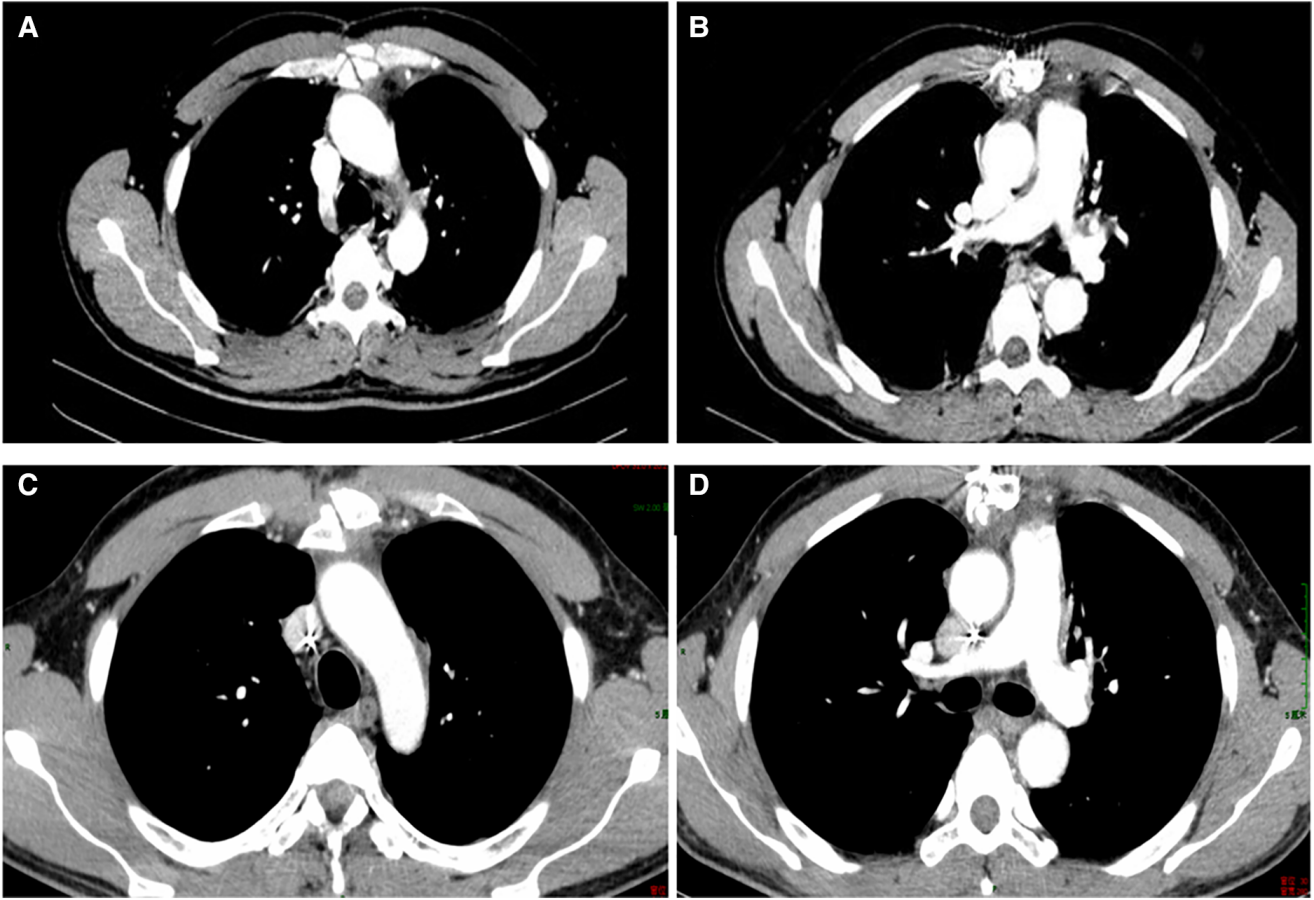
CT in the 1-month follow-up (A,B); CT in the 7-month follow-up (C,D). CT, computed tomography.
